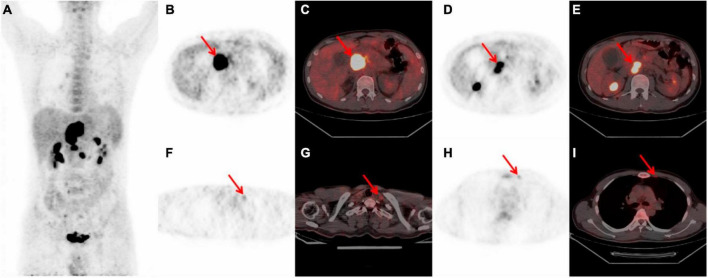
Whole body ^18^F-FDG PET/CT scan of a 31-year-old male with PPL. (A) MIP showed a hypermetabolic mass in the superior abdominal and peripheral lesions. And mild hypermetabolic of FDG were observed in the thoracic spine. (B, C) A 5.5 × 5.4 cm high FDG-avid mass at the head and neck of the pancreas with SUVmax 22.0 (red arrow). (D,E) Hypermetabolic lymph nodes in the posterior of pancreatic region (red arrow). (F,G) Left clavicular region lymph node with mild FDG hypermetabolism (red arrow). (H, I) Suspicious mild hypermetabolism within the left internal mammary lymph nodes (red arrow). PPL, primary pancreatic lymphoma; ^18^F-FDG PET/CT, ^18^F-fluorodeoxyglucose positron emission tomography/computed tomography; MIP, maximum intensity projection; SUVmax, maximal standardized uptake value; CT, computed tomography.
